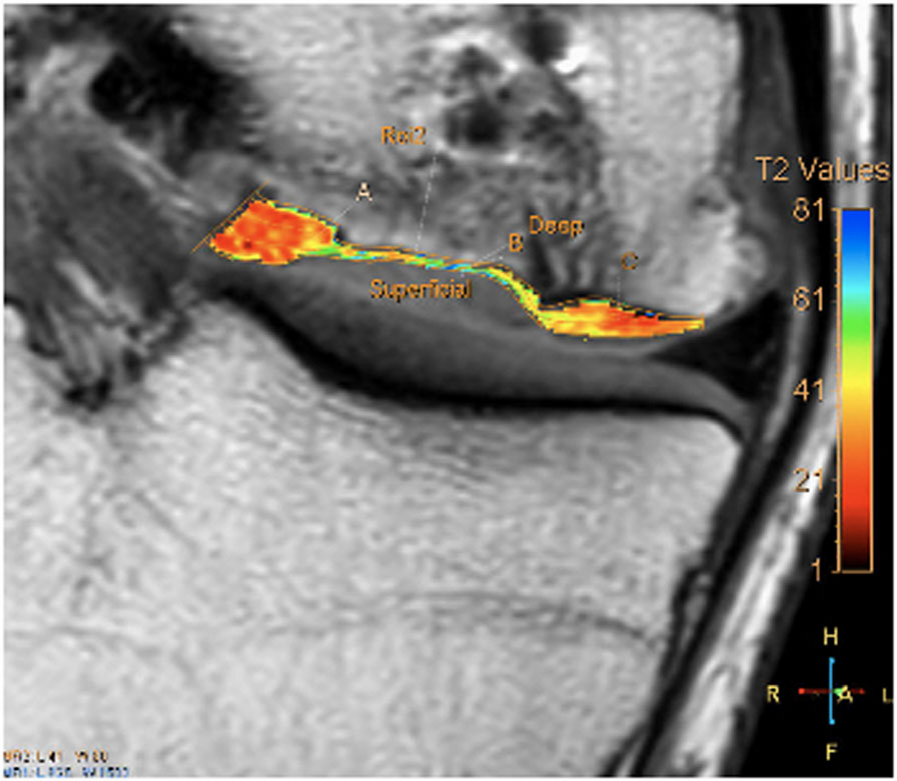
Pre-Treatment MRI T2 mapping of the medial compartment of the knee indicating elevated hydration of the cartilage in the area surrounding the OCD and also of the thin layer of tissue which partially covered the defect
